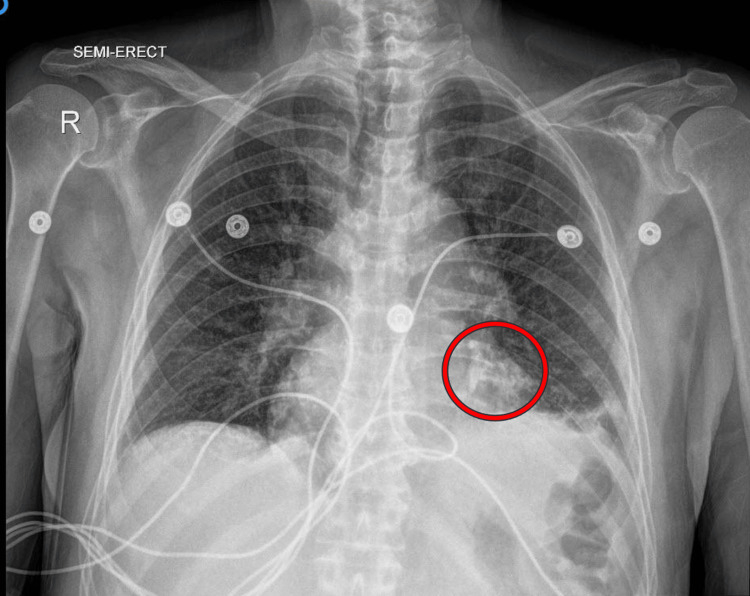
Postero-anterior (PA) chest radiography view Left basilar subsegmental atelectasis with no focal pulmonary consolidations or pleural effusions. Cardiomediastinal silhouette is within normal limits in size. Linear hyperdensity overlying the heart likely represents coronary artery calcifications (indicated by the red circle).
